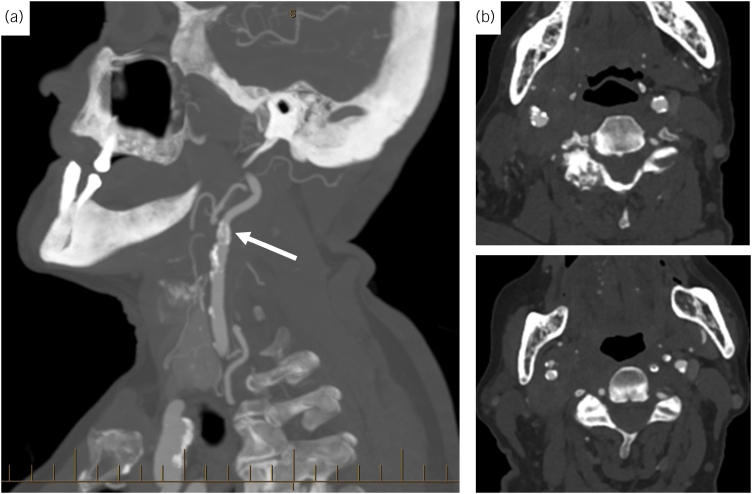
(a) Sagittal view of the computed tomography arteriography (CTA) demonstrating no evidence of an internal carotid artery pseudoaneurysm (white arrow). (b) Axial views of the CTA demonstrating a normal diameter right common carotid and internal carotid artery with no pseudoaneurysm seen.
